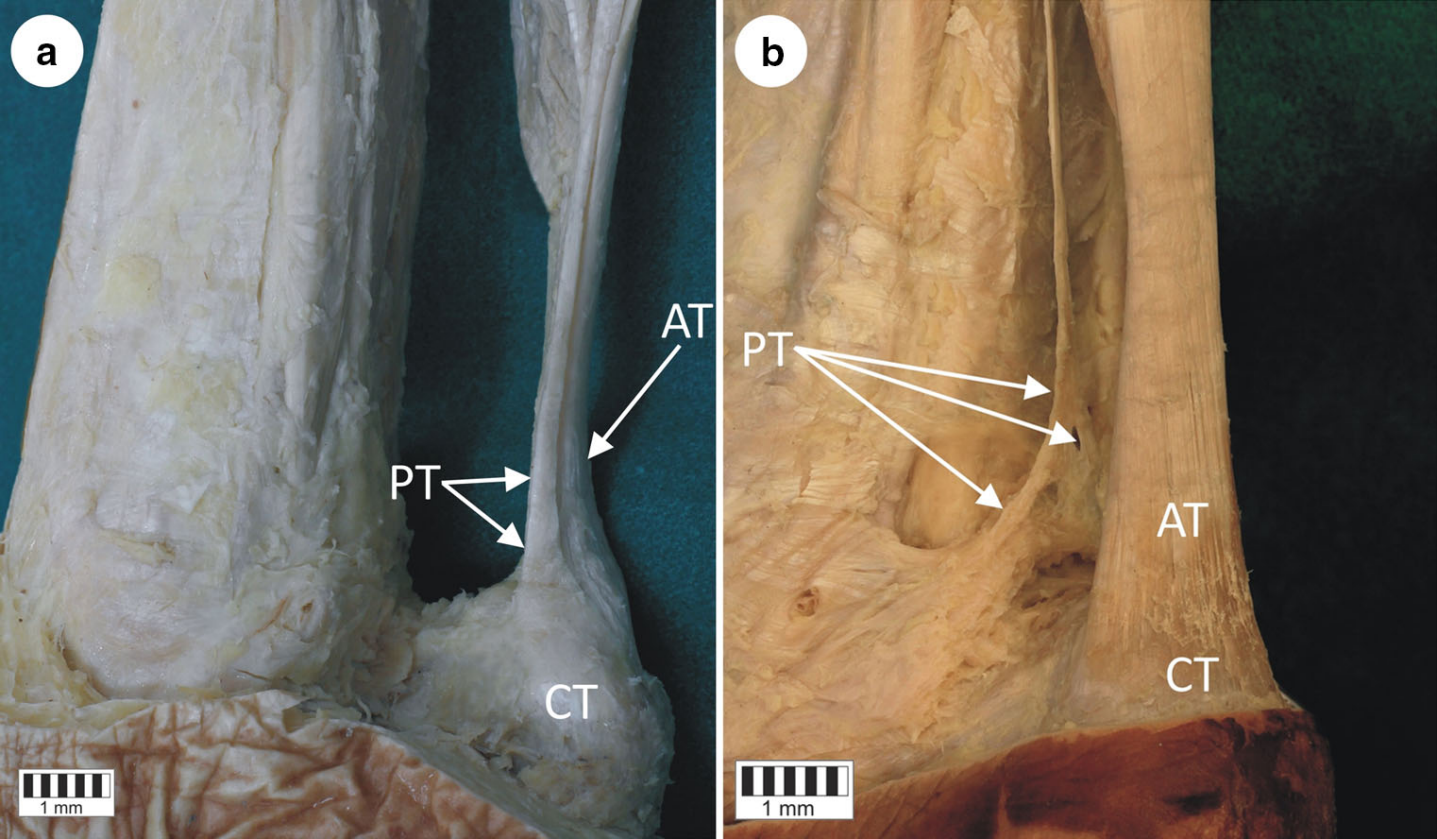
Tendon of the plantaris muscle. a Type 1 of plantaris tendon insertion to the calcaneal tuberosity (the most common). Medial view of the right leg. The course of the plantaris muscle tendon visible on the medial part of the calcaneal tendon. b Type 4 of the plantaris tendon insertion (the less common). Posteromedial view of the right leg. The figure shows the atypical insertion of the plantaris tendon to the deep fascia of the leg. The lack of the insertion located on the calcaneal bone is visible. *AT* Achilles tendon, *CT* calcaneal tuberosity, *PT* plantaris tendon
